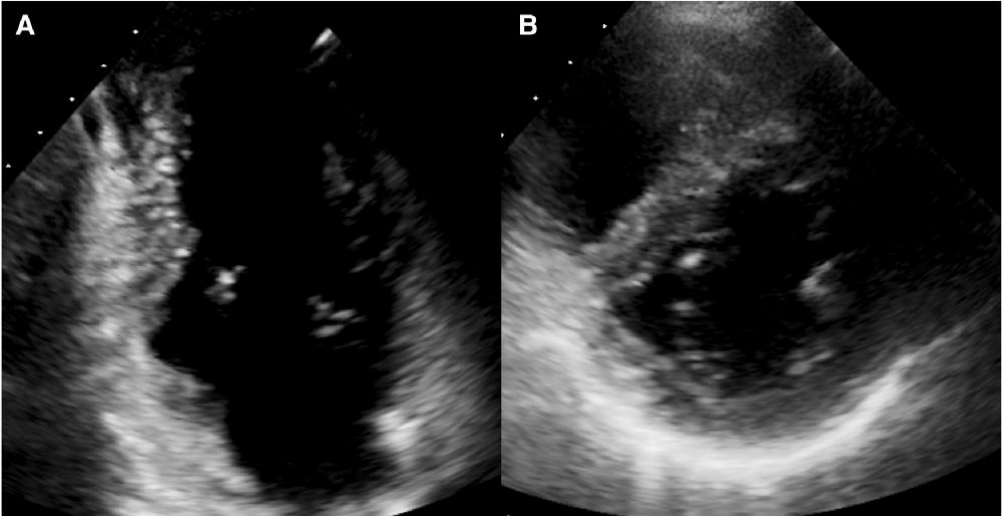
Patient 2, transthoracic echocardiogram: (*A*) apical two-chamber view showing diverticulum of the basal portion of inferior wall (length 3.0 cm, depth 1.9 cm). (*B*) Parasternal short axis view.
